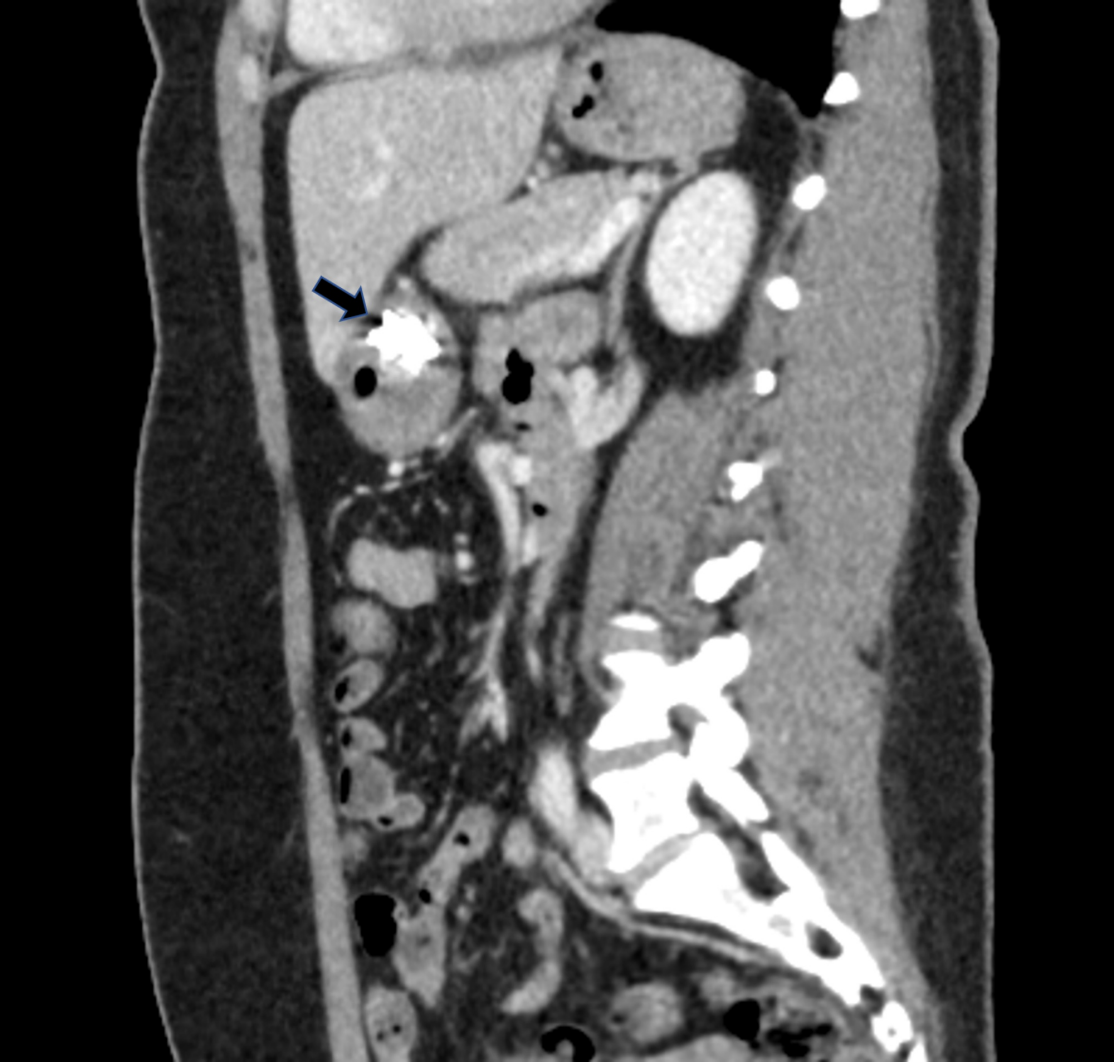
Abdominal CT scan revealing intact Padlock clip two years later
